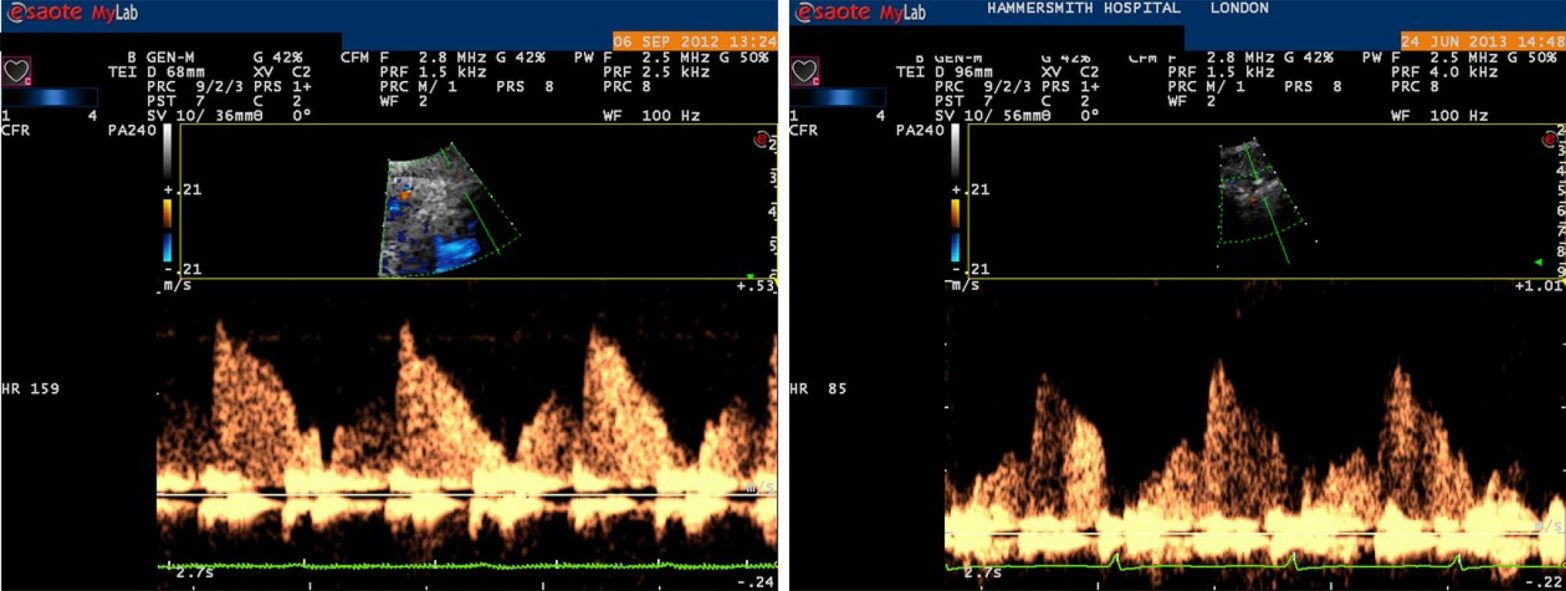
Example of non-invasively obtained coronary flow using transthoracic echocardiography
